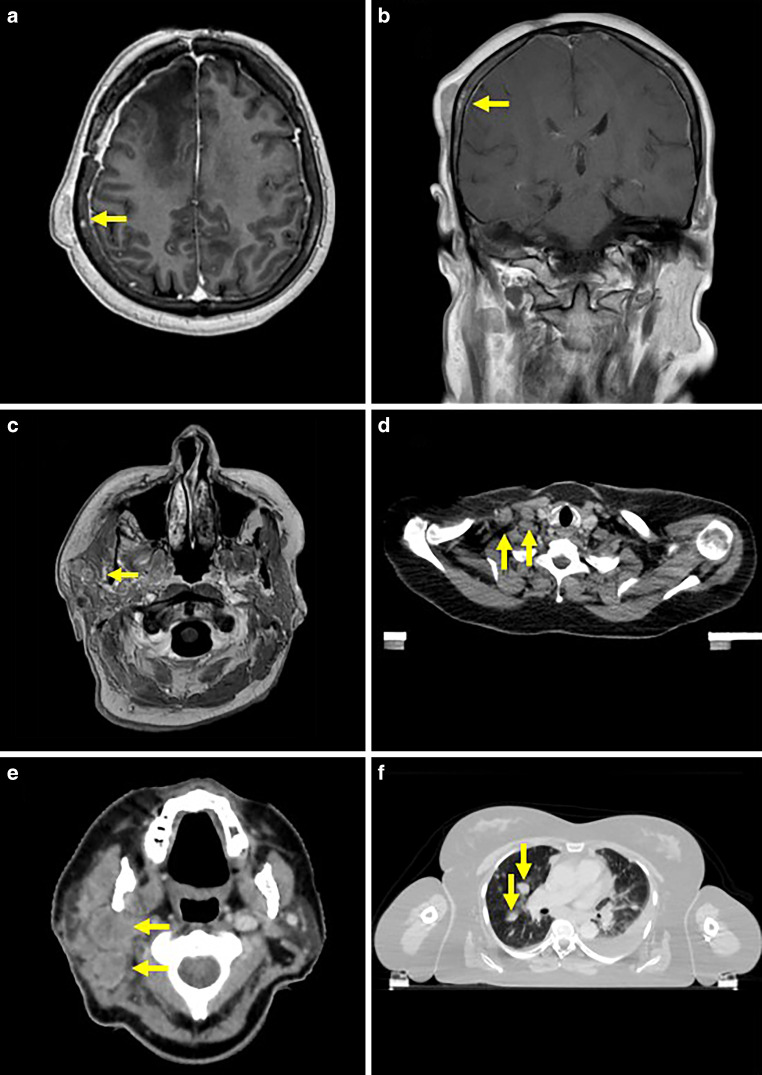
Imaging of systemic spread: MRI scans of the second glioblastoma manifestation in the right temporoparietal skull (a, b; arrow) and painful metastases in the right parotid (c; arrow) and CT scans of enlarged cervical lymph nodes (d, e; arrows) and pulmonary metastases (f; arrows)
